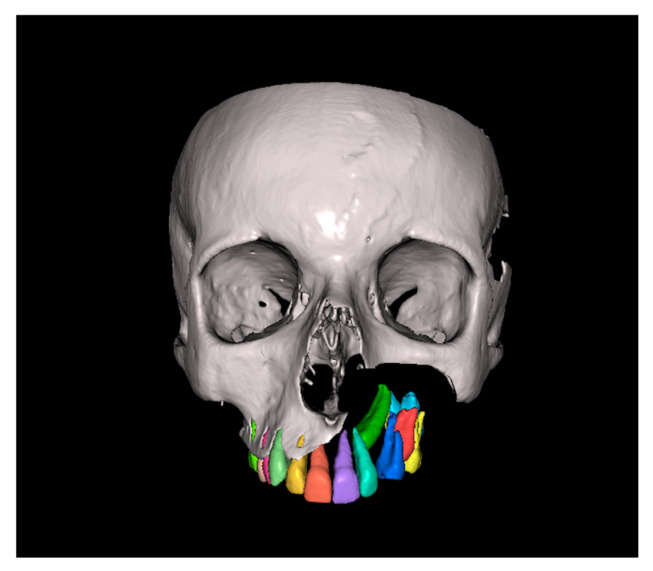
Initial CBCT after tooth segmentation.
